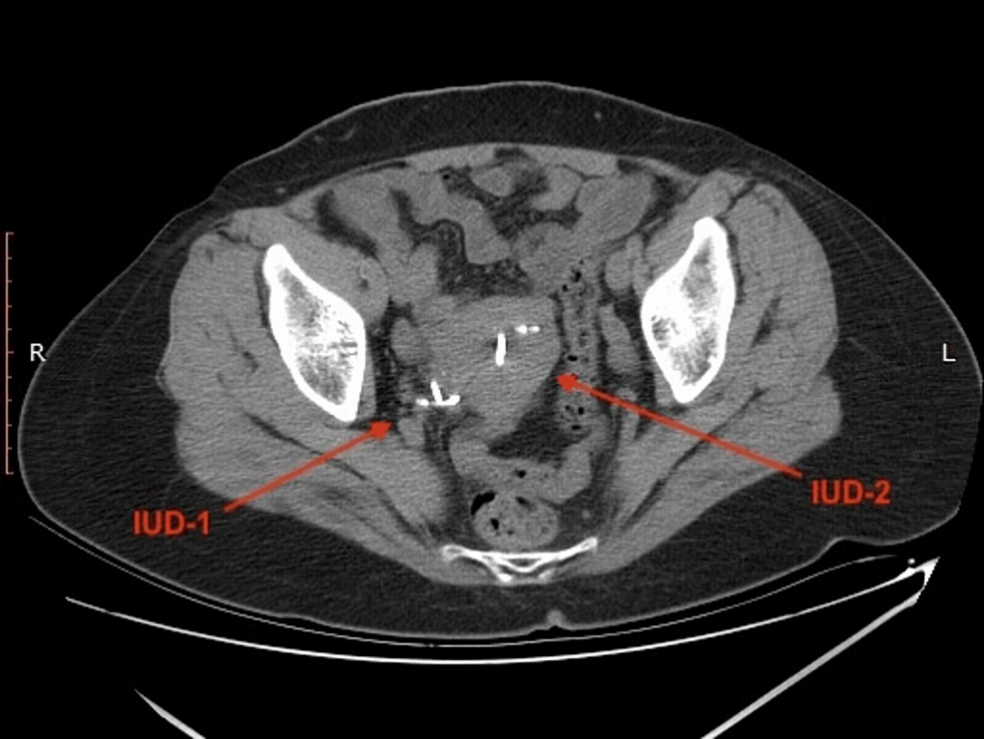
On CT examination, IUD-1 was observed in the distal ureter and IUD-2 was seen in the uterus. IUD: intrauterine device.
